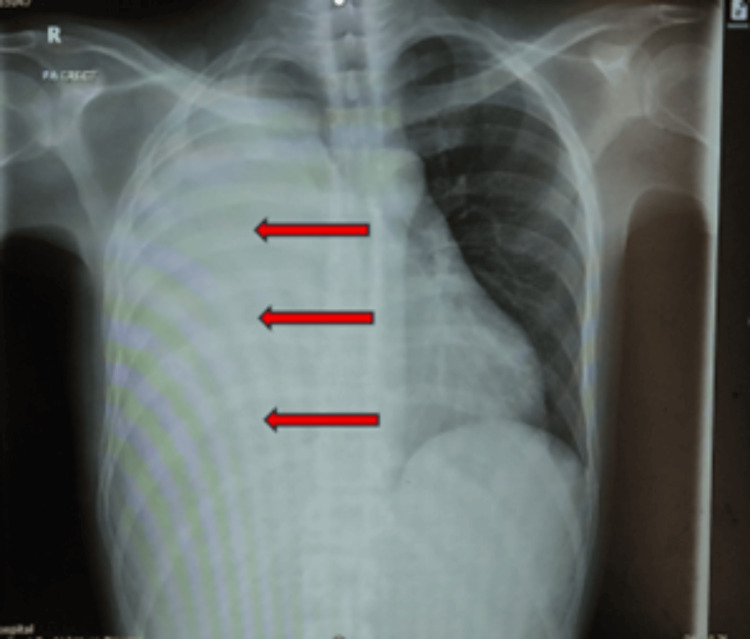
Initial posteroanterior (PA) erect chest X-ray demonstrating near-complete opacification of the right hemithorax, consistent with a massive pleural effusion
